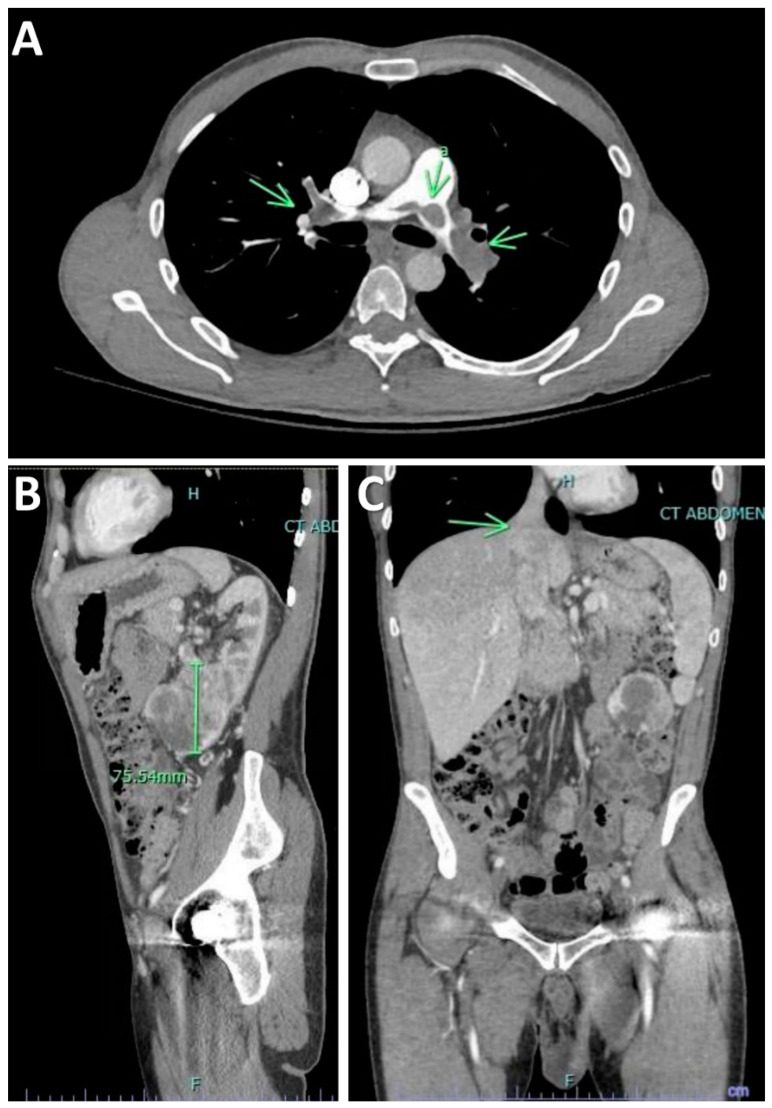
Imaging studies. (A) Computerized tomography angiogram (CTA) of the chest was notable for extensive filling defects consistent with saddle pulmonary embolism. (B) Abdominal CT scan revealed 6.5 × 8.0 × 7.6 cm heterogenous mass at the left inferior pole abutting Gerota’s Fascia, concerning for renal cell carcinoma, clear cell type. (C) Tumor thrombus was also seen in the left renal vein and inferior vena cava, extending to the level of the diaphragm.
